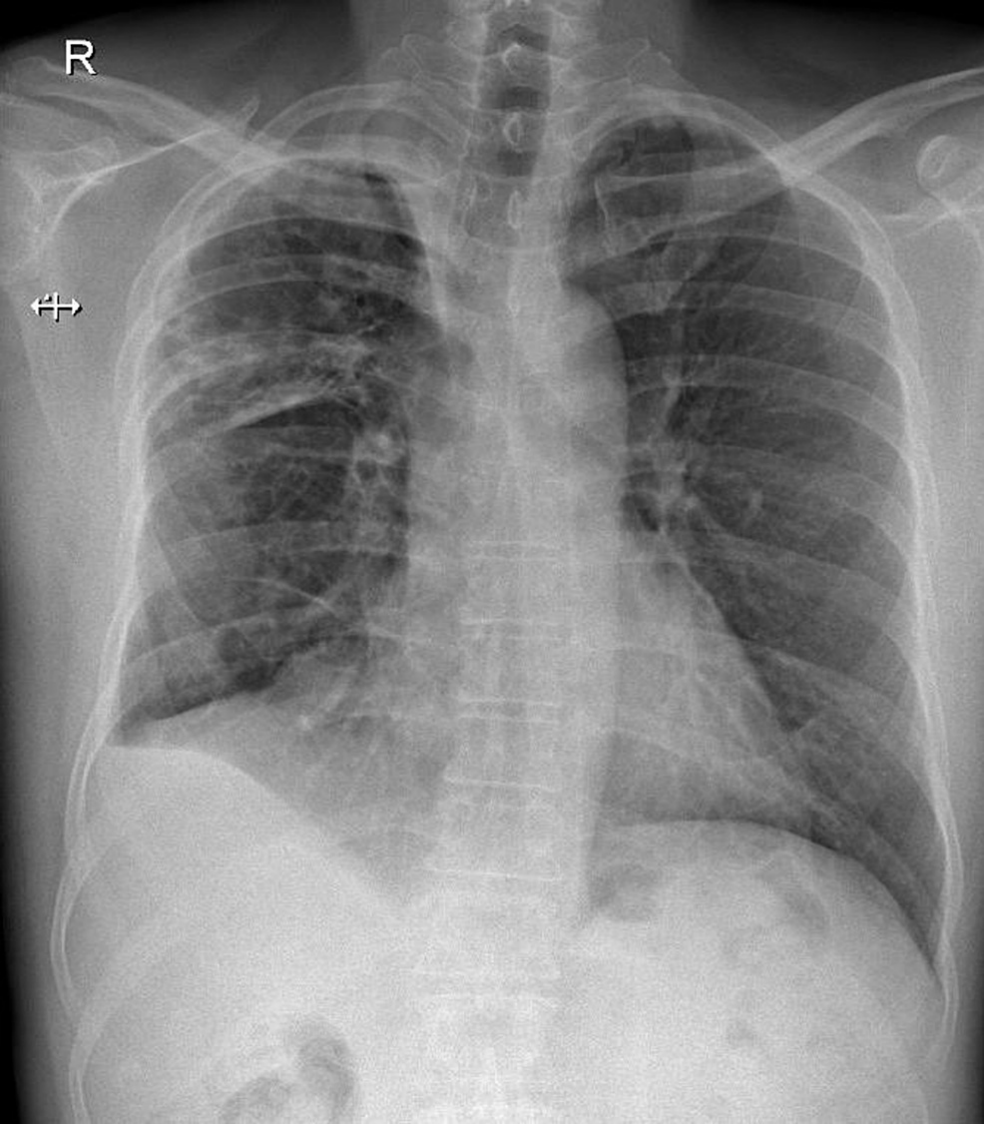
Chest radiograph (posteroanterior erect view) taken at presentation. No abnormal findings were noted.
